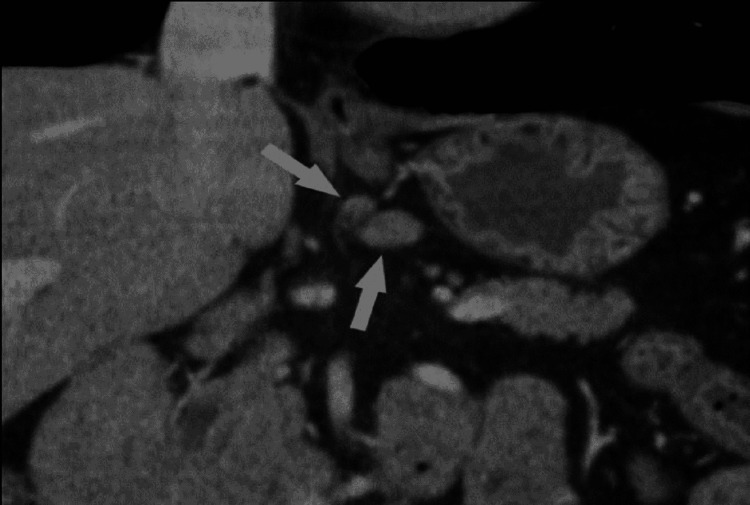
Abdominal computed tomography scan showing a mass in the stomach
